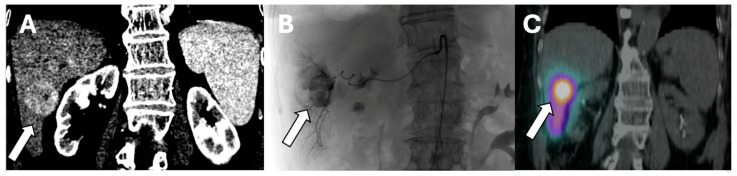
64-year-old male patient with a right hepatic HCC who underwent Y-90 radiation segmentectomy treatment. (A) Pre-procedure CT demonstrating arterially enhancing right hepatic lobe HCC (arrow), (B) intraprocedural arteriogram demonstrating the lesion (arrow) on fluoroscopy during the Y-90 treatment session, and (C) post-treatment SPECT CT demonstrating Y-90 activity deposited in the tumor (arrow).
